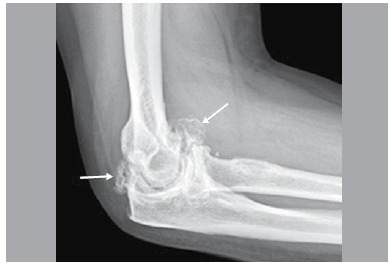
Radiographic alterations grade 4. Articular destruction and loose bodies (arrows).
